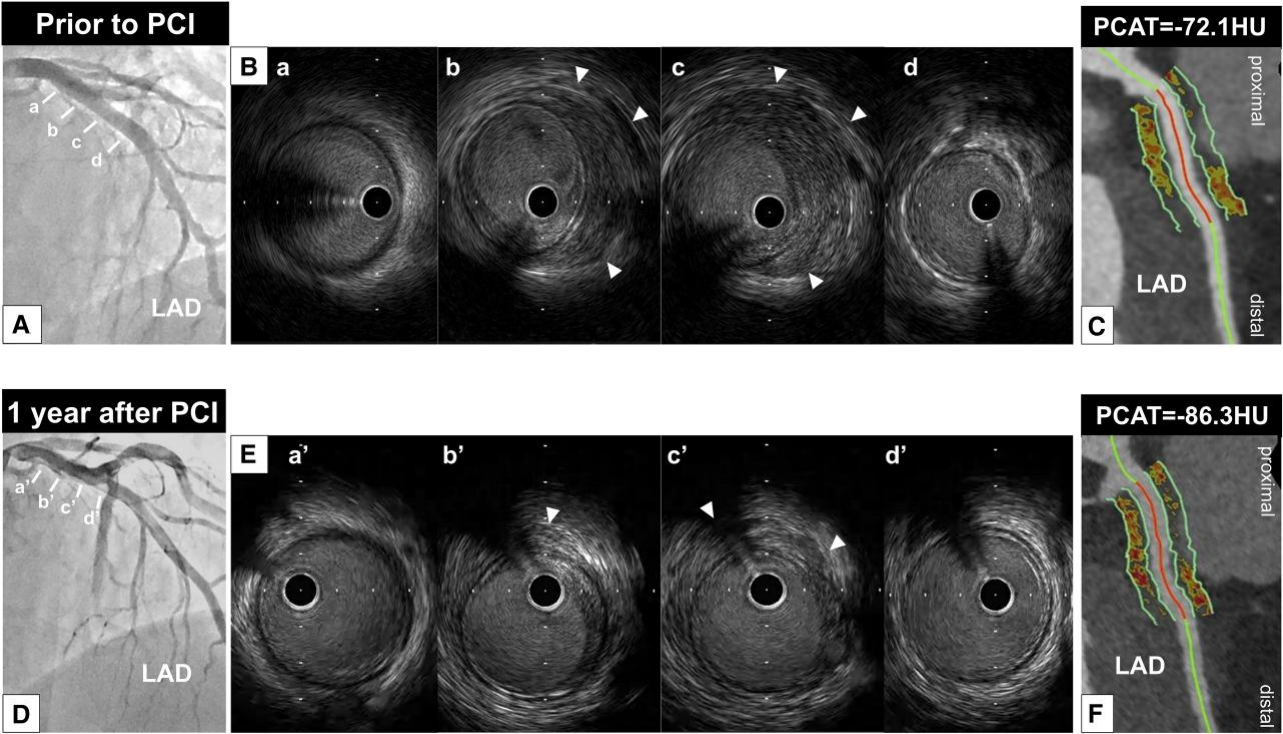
(*A*) No significant stenosis was observed in the left anterior descending artery. (*B*) Intravascular ultrasound imaging revealed thickening of the adventitia (white arrowheads) at the proximal segment of the left anterior descending artery. (*C*) Coronary computed tomography angiography imaging 12 days after primary percutaneous coronary intervention demonstrated soft tissue proliferation surrounding the proximal segment of the left anterior descending artery. The average pericoronary adipose tissue attenuation at this segment was −72.1 HU. (*D*) Coronary angiographic features remained unchanged 1 year after therapy. (*E*) A decrease in the thickness of the adventitia (white arrowheads) was observed on intravascular ultrasound imaging. (*F*) Coronary computed tomography angiography imaging demonstrated a reduction in soft tissue volume, and pericoronary adipose tissue attenuation decreased to −86.3 HU. HU, Hounsfield units; IVUS, intravascular ultrasound; LAD, left anterior descending artery; PCAT, pericoronary adipose tissue attenuation; PCI, percutaneous coronary intervention.
